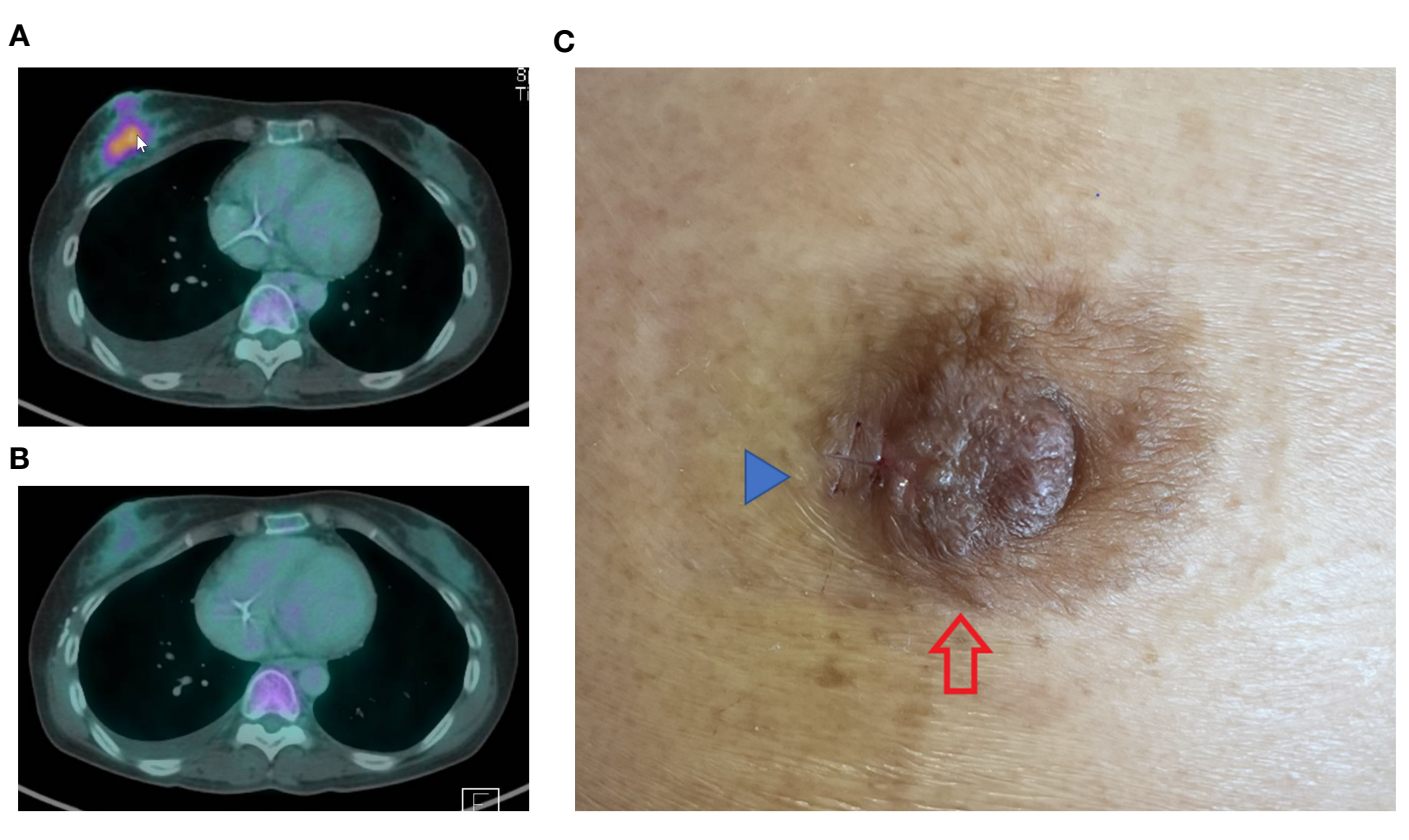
PET CT images dated 16/10/2023 (A) depict a highly metabolic right breast lesion. The cancer exhibited significant response following only 3 cycles of combination chemotherapy incorporating Trastuzumab (TCH regimen), as evidenced by the image from 18/12/2023 (B). The nipple (C) showed a rash at the lateral aspect of the right nipple areolar complex (red arrow), clinically mimicking Paget disease. A small surgical scar (blue arrow) is observed at the lateral edge of the rash, attributed to a recent incisional skin biopsy.
